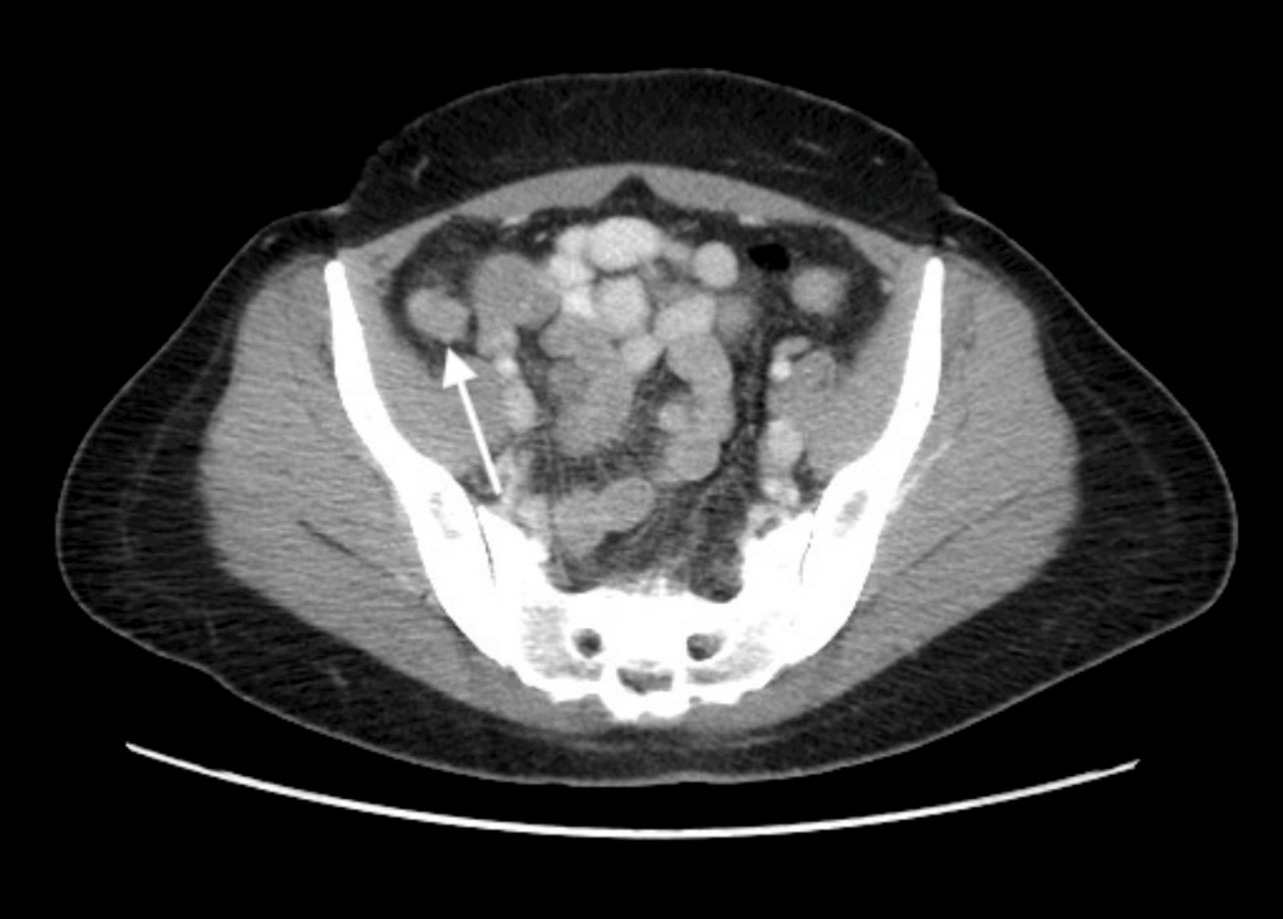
Cross-sectional view of CT of the abdomen and pelvis showing mild thickening and inflammatory changes of the appendix without evidence of perforation.
